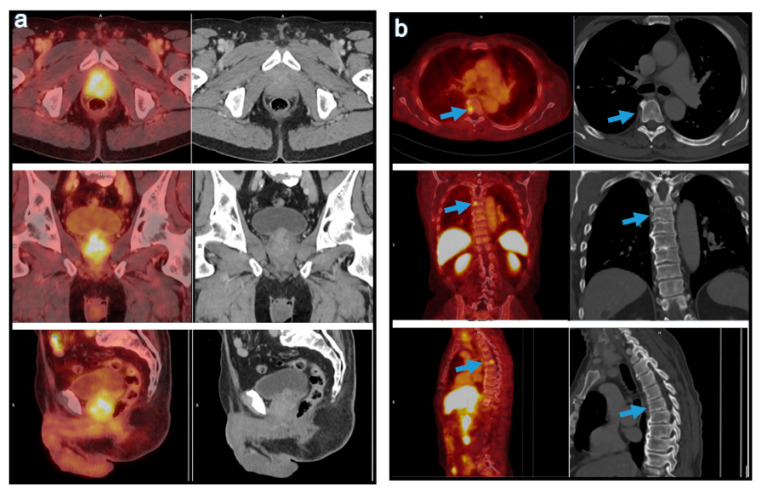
Primary staging of a treatment-naïve, 70-year-old patient with PCa metastasizing to the 5th and 9th thoracic vertebrae, using [^18^F]-PSMA PET–CT. The PSA value at the time of examination was 7.8 µg/L. (a) The PSMA-positive primary tumor (SUVmax 6.4). (b) The PSMA-positive bone metastasis in T5 (SUVmax 3.7).
